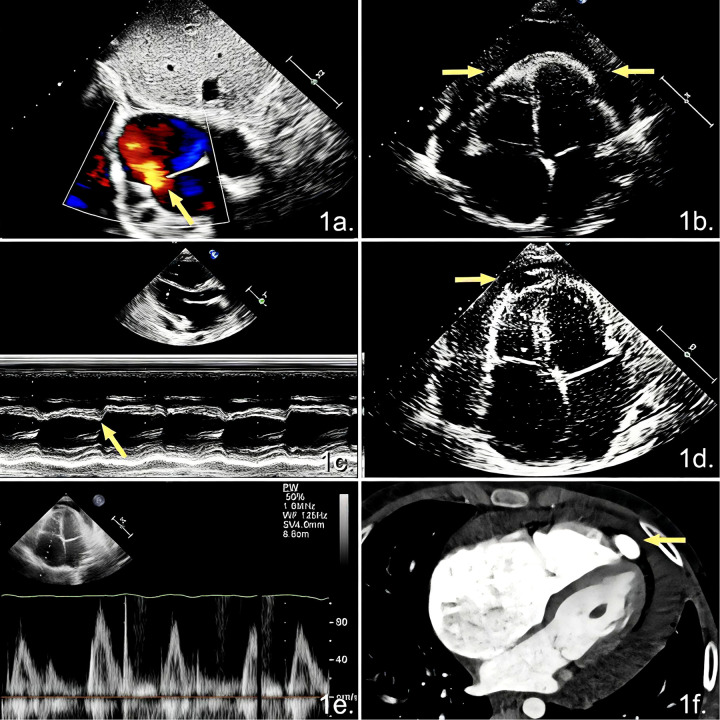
Preoperative TTE and contrast-enhanced cardiac CT images. (a) Subcostal bicaval view demonstrating an ASD with a left-to-right shunt. (b) Apical four-chamber view showing pericardial thickening associated with pericardial effusion. (c) M-mode tracing from the parasternal long-axis view revealing septal bounce in early diastole. (d) Apical four-chamber view showing a cystic structure at the right ventricular apex. (e) Pulsed-wave Doppler demonstrating >25% respiratory variation in early diastolic tricuspid valve inflow velocity. (f) Contrast-enhanced cardiac CT showing a contrast-filled outpouching at the right ventricular apex.
